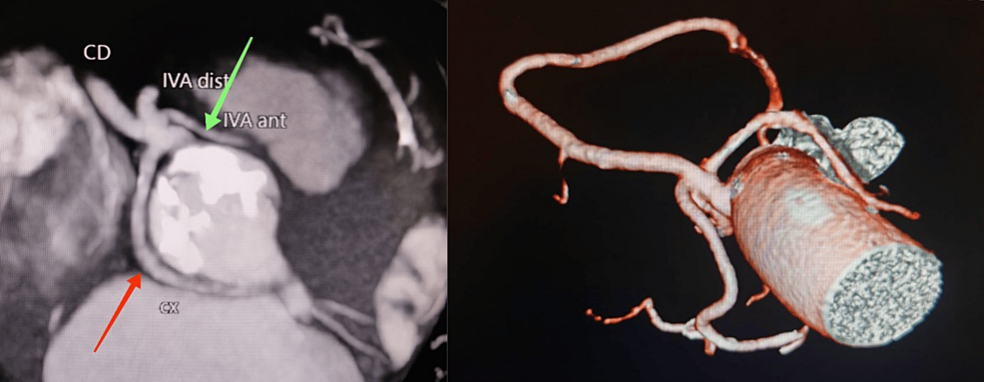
Multidetector computed tomography showing all three major coronaries arising from the right sinus via common ostium. The proximal LAD has an interarterial course (Green arrow). The Cx has a retroaortic course (Red arrow). LAD: left anterior descending artery; Cx: circumflex
